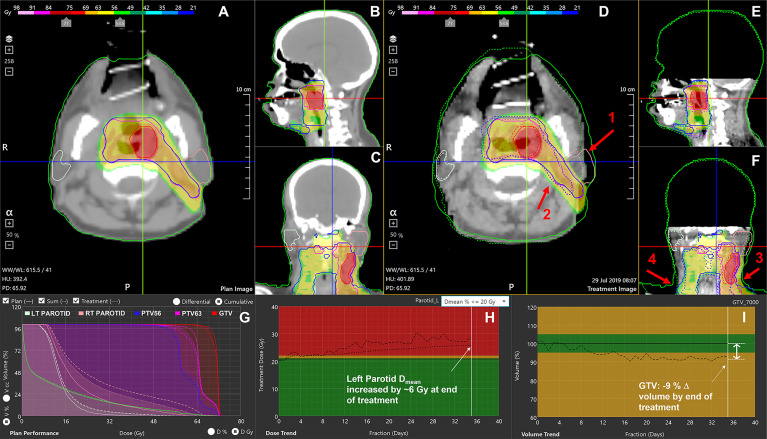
RTapp software user interface. (A–C) Initial plan CT, structures, and 3D dose. (D–F) The final fraction’s CBCT with plan structures (solid line), deformed contours to current day’s position (dotted line), and initial 3D dose. (G) DVH showing the plan dose (solid line), delivered dose up to the current fraction (DVH_sum_, dotted line), and day of treatment dose scaled to full treatment (DVH_day_, dashed line). (H) Trend for parotid’s mean dose; (I) tumor volume regression throughout the treatment. The red arrows indicate notable effects of RT and weight loss on the left parotid (1), PTVs (2), external neck contour (3), and inconsistent shoulders repositioning (4).
